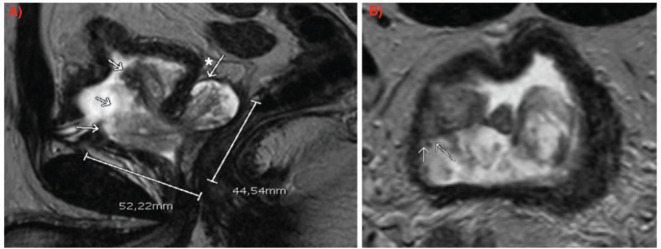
MRI (a): Sagittal slice: arrows indicating intravesical growth of the lesion. The asterisk with the arrow marks the involvement of the left seminal vesicle. (b): Axial slice: arrows indicating tumour contact with bladder wall.
